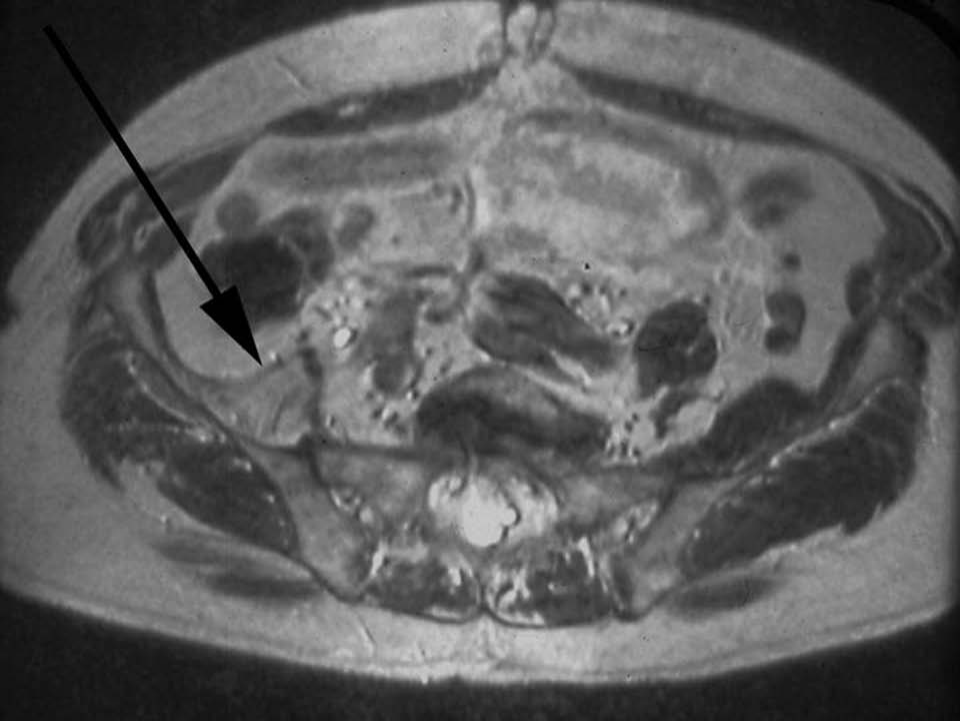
An axial T2 MRI showing the fatty atrophy and retraction of the right ilio-psoas tendon (arrow) all the way to the level of the sacro-iliac joint.
